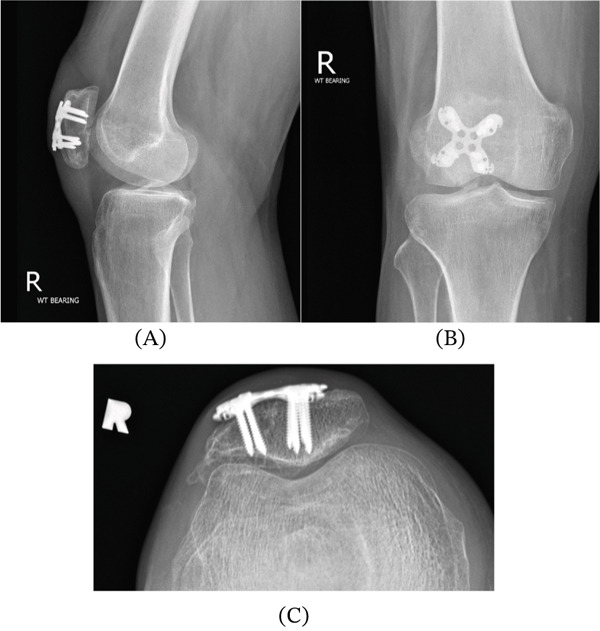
Radiographs demonstrating (A) lateral, (B) AP, and (C) sunrise views at 5 months postoperative with healed fracture and intact hardware.
